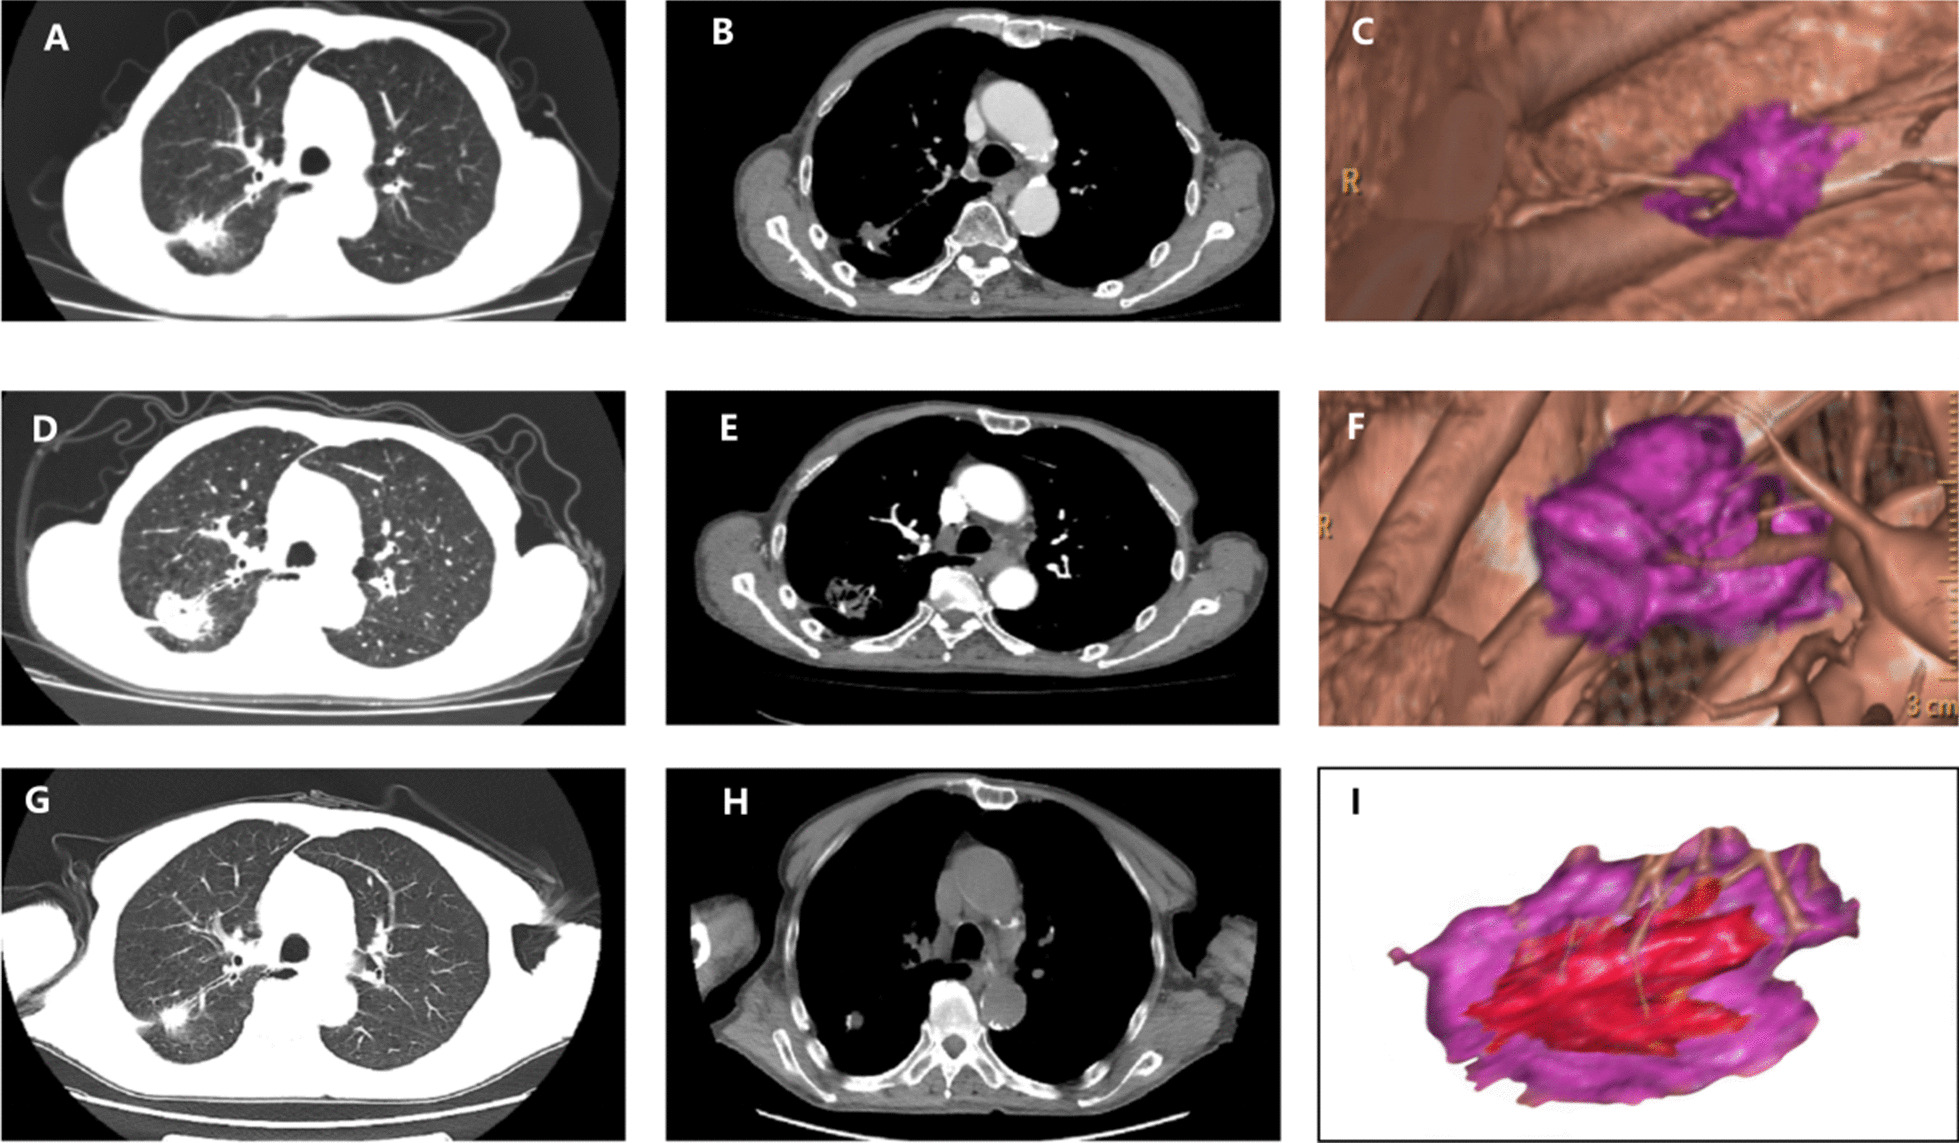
a, b One lesion was clearly seen in the lung window and mediastinal window, which was pathologically confirmed as squamous cell carcinoma by biopsy. c 3D reconstruction revealed the size and margin of the tumor (purple color). d, e 2 days after ablation, CT showed no pneumothorax and pleural effusion, and the ablation zone was visible. f 3D reconstruction revealed the ablation zone and spatial relationships of the tumor (purple color). g, h 10 months after MWA, the ablative zone shrink and fibrosis had developed. i The images of pr-ablation (c) and postablation (f) were fused and displayed as (i). Quantitative measurement shown the minimal ablative margin was > 5 mm
